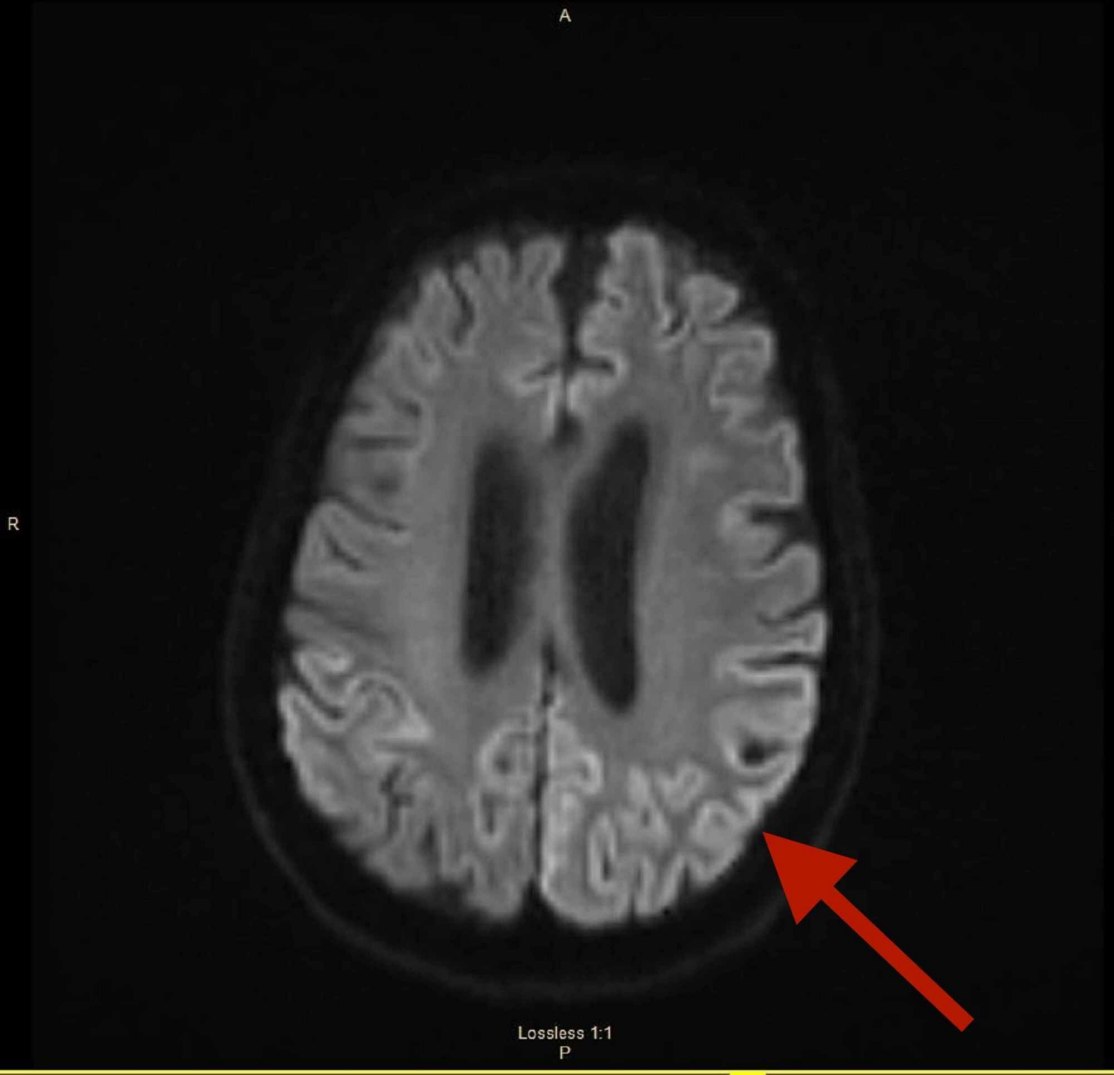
MRI brain shows a signal hyperintensity of cortical gyri, characteristically known as the cortical ribbon sign
